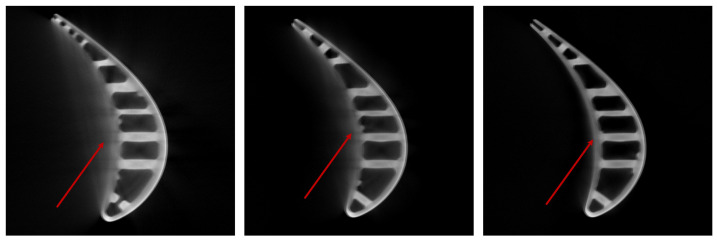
ICT images of turbine blades affected by different degrees of artifacts—the degree decreases from left to right. Severe artifacts have seriously affected the measurement of the wall thickness of blades in CT images.
